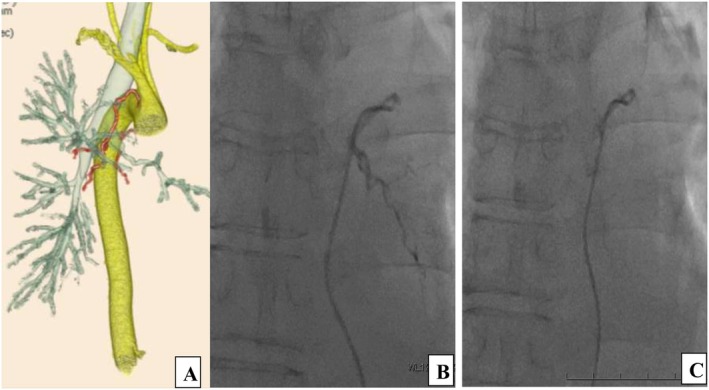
Three dimensional CT image shows an aberrant artery branching from the brachiocephalic artery of the right bronchial artery. The gelatin sponge embolized the left bronchial artery. Bronchial angiography after BAE reveals disappearance of vascular shadows. (A) Angiography image. (B) Before BAE. (C) After BAE.
